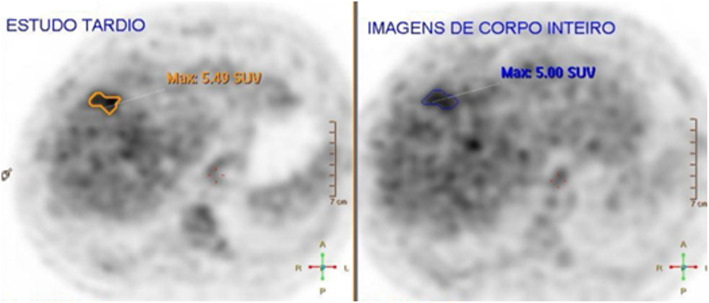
Position emission tomography/computed tomography showing irregular liver uptake and an ill-defined area with higher fluorodeoxyglucose uptake in liver segments VIII/IV, with maximal SUV=5 at initial phase (right), and SUV=5.5 at the delayed phase (left)
